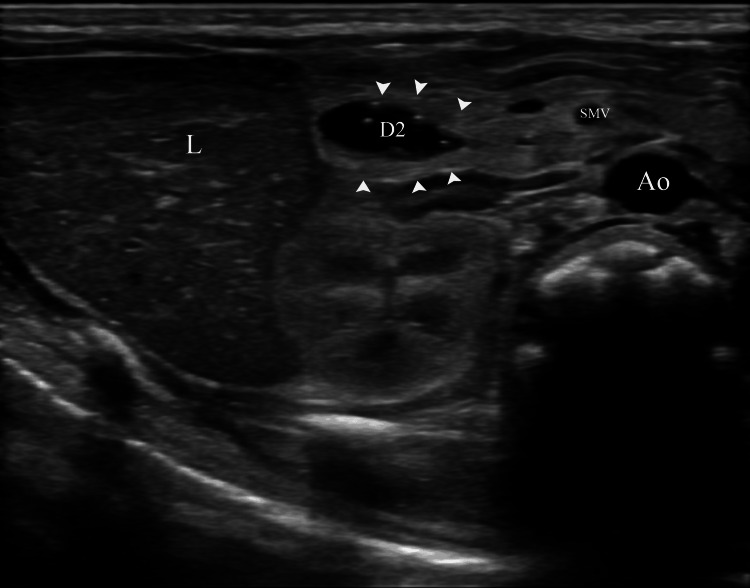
Gray-scale ultrasound image raising suspicion for an annular pancreas Gray-scale transverse plane reveals encircled fluid with strong internal echoes, which is encased by hyperechogenic tissue (arrowheads) of the pancreatic head. Internal echoes account for intraluminal air in the duodenum. Ao, Aorta; D2, Descending duodenum; L, Liver; SMV, Superior mesenteric vein
